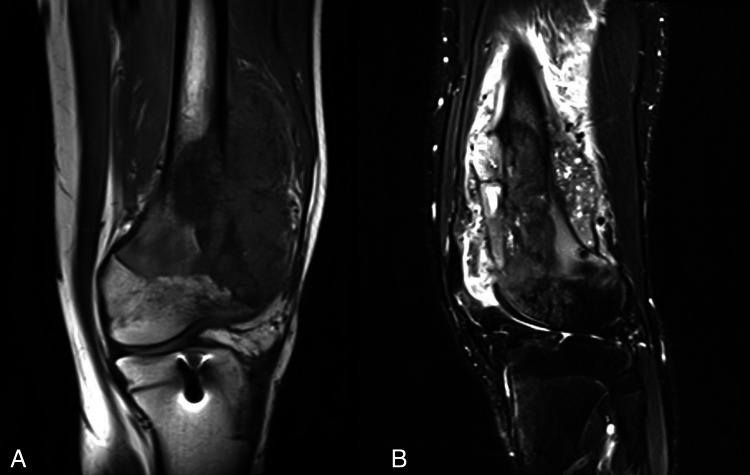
(a) T1-weighted coronal and (b) T2-weighted fat-suppressed sagittal magnetic resonance images of the left knee of a 16-year-old boy (patient four). Images show a T1-weighted hypointense and T2-weighted heterogenous lobulated mass involving the distal femoral metaphysis and lateral condyle after anterior cruciate ligament reconstruction.
